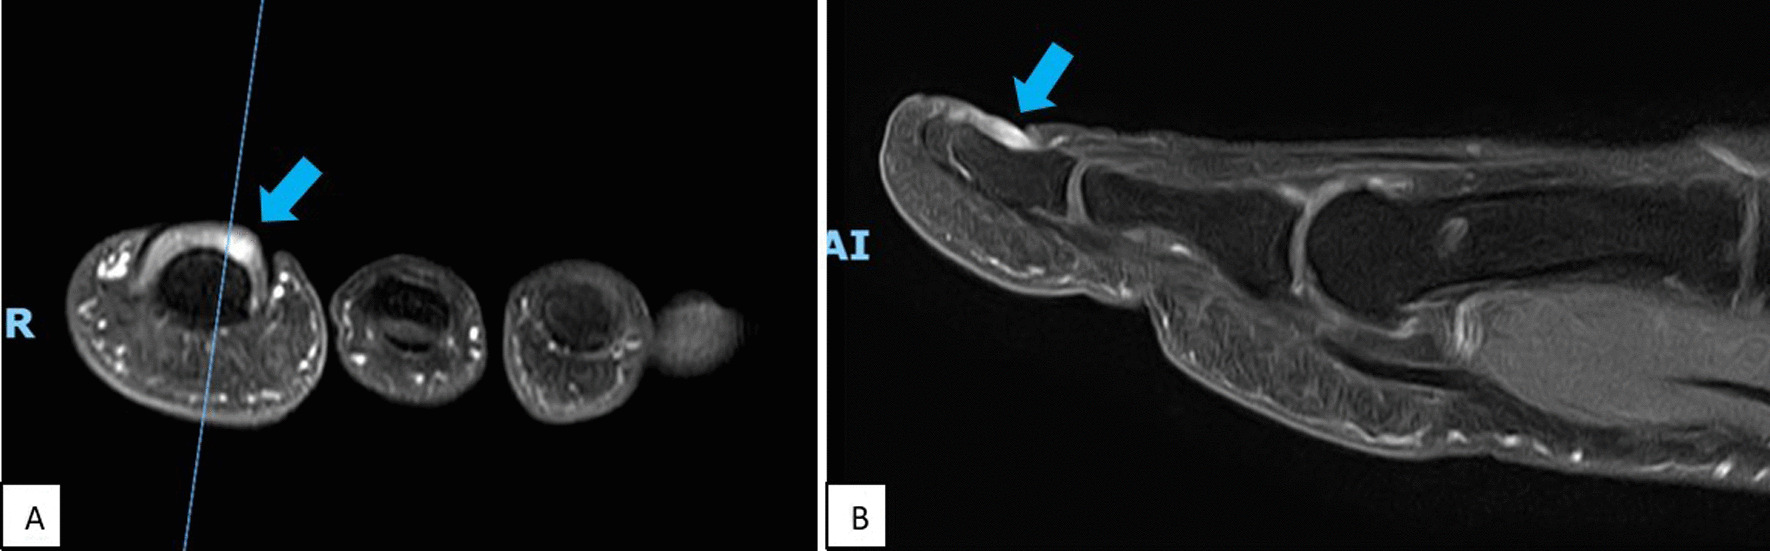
T2-weighted coronal A and sagittal B cuts of the patient’s left big toe. Contrast-enhancing nodule (blue arrows) noted in the proximal lateral subungual region with no obvious cortical erosion of the distal phalanx
